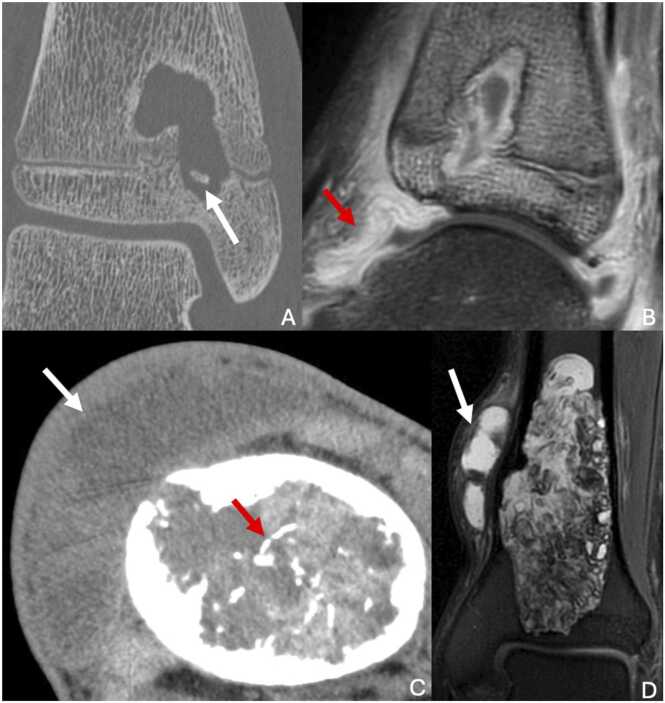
A,B. Osteomyelitis with Brodie’s abcess of the distal metaphysis of right tibia in a 16-year-old male adolescent presenting with inflammatory pain in the right ankle for three months, with a biological inflammatory syndrome. C,D. Bone hydatid cyst of the distal third of left tibia in a 43-year-old man from Mali with 18 months of left leg pain (different patient). A. CT image in the coronal plane showing an osteolytic lesion with bone sequestration (white arrow), centered in the metaphysis. B. T1-weighted fat-suppressed contrast-enhanced MR image in the sagittal plane showing peripheral enhancement of the Brodie’s abcess, adjacent osteomedullar enhancement in relation to bone edema and an important synovitis tibiotalar joint (red arrow). C. CT image in soft tissue reconstruction in the axial plane showing heterogenous content of an osteolytic lesion, with thin central linear calcifications (red arrow), and extension to soft tissue (white arrow). D. T2-weighted fat-suppressed MR image in the coronal plane highlighting multiple daughter vesicles inside the large bone vesicle associated with pluriloculated soft-tissue collection (white arrow).
